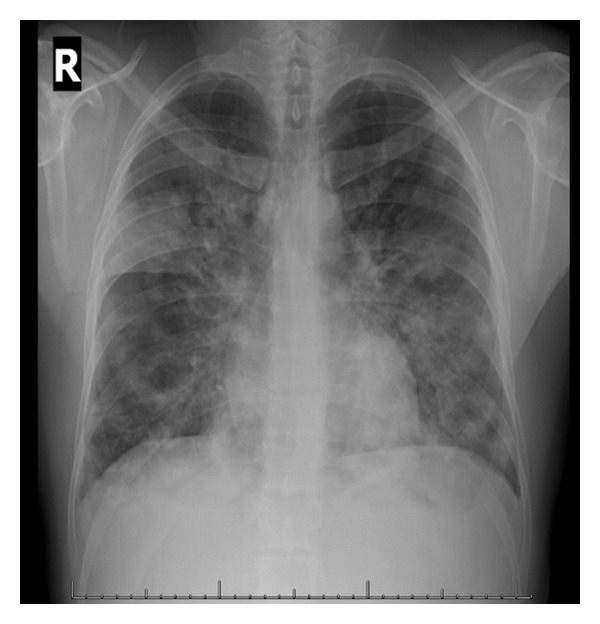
Initial chest X-ray at the ICU, showing diffuse bilateral cotton-like infiltrates.
